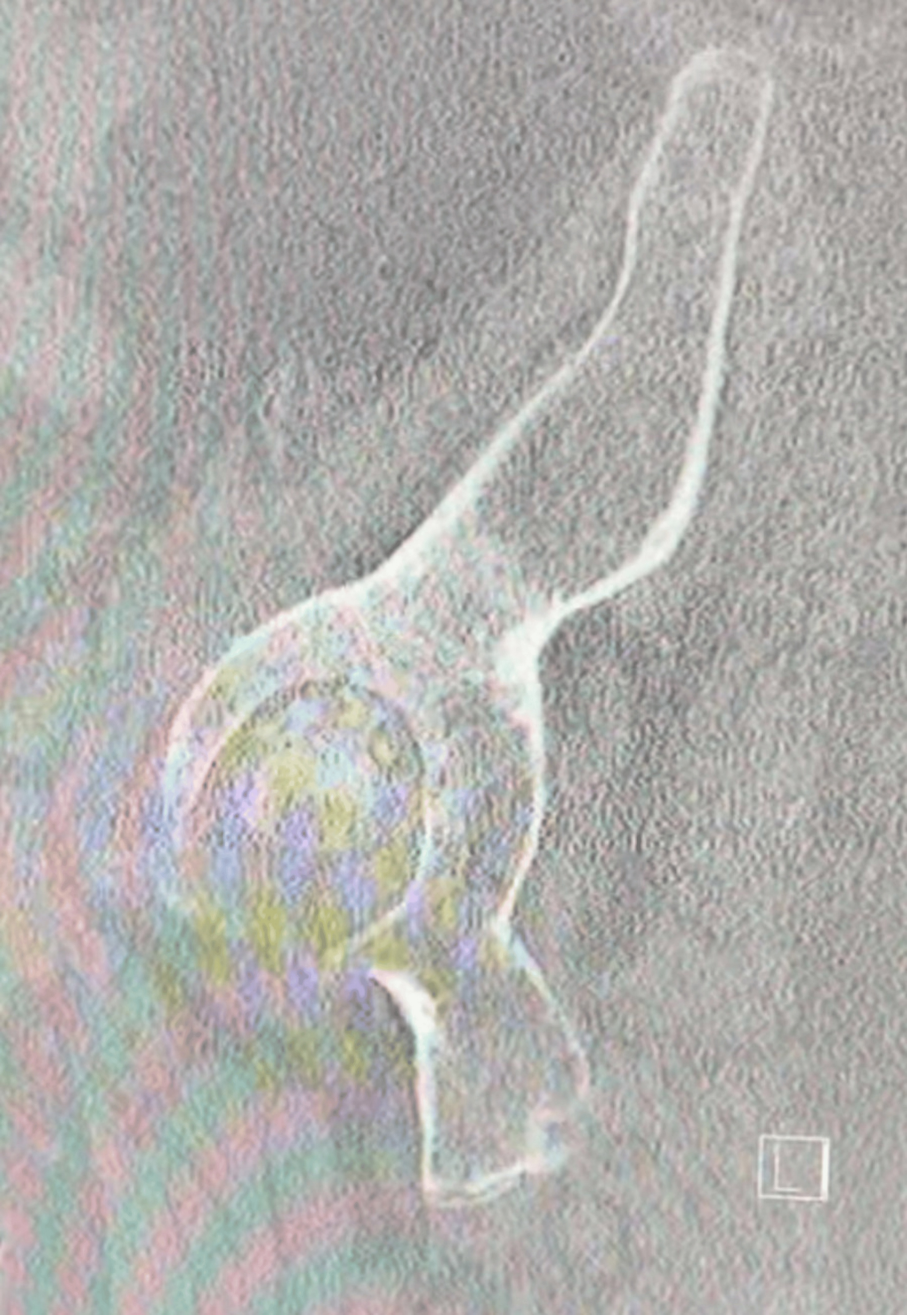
Preoperative sagittal CT of the hip
